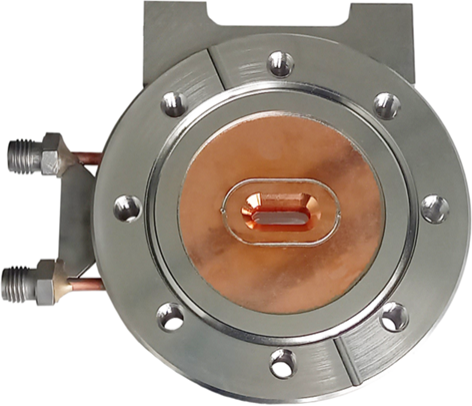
CVD diamond window for hard X-ray front ends.
